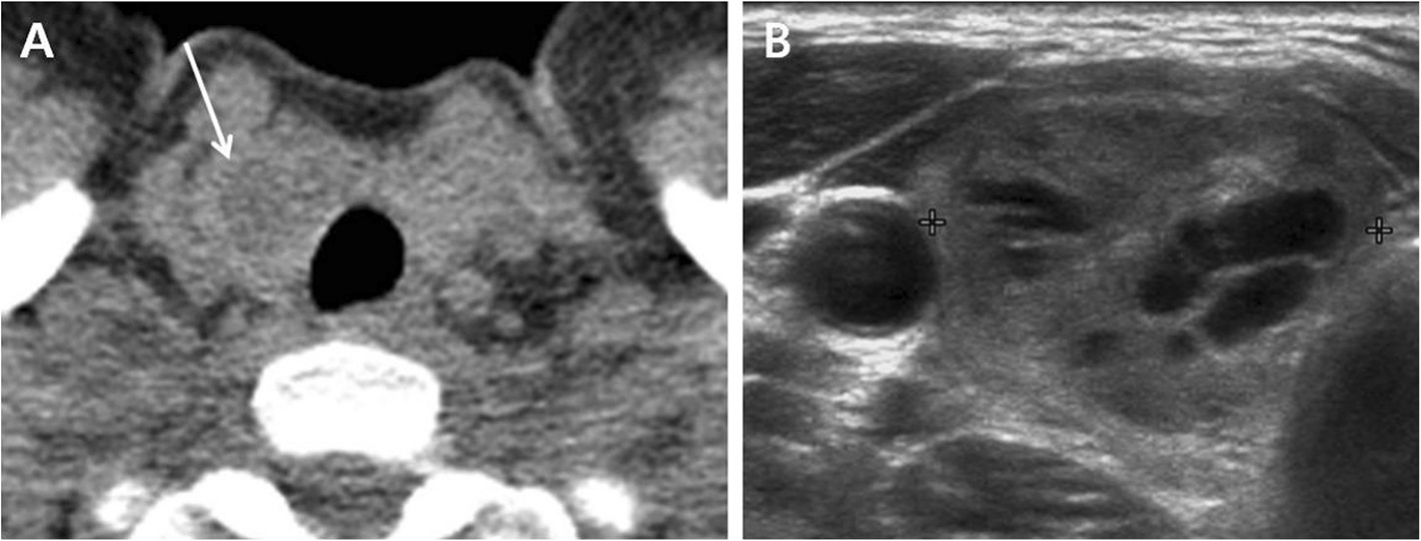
A 49-year-old woman with an adenomatous goiter. (A) Low-dose helical CT scan demonstrated a 21 mm hypodense nodule (arrow) without calcification in the right thyroid lobe. (B) Transverse US image of the right thyroid lobe showed a mixed solid and cystic nodule (calipers).
